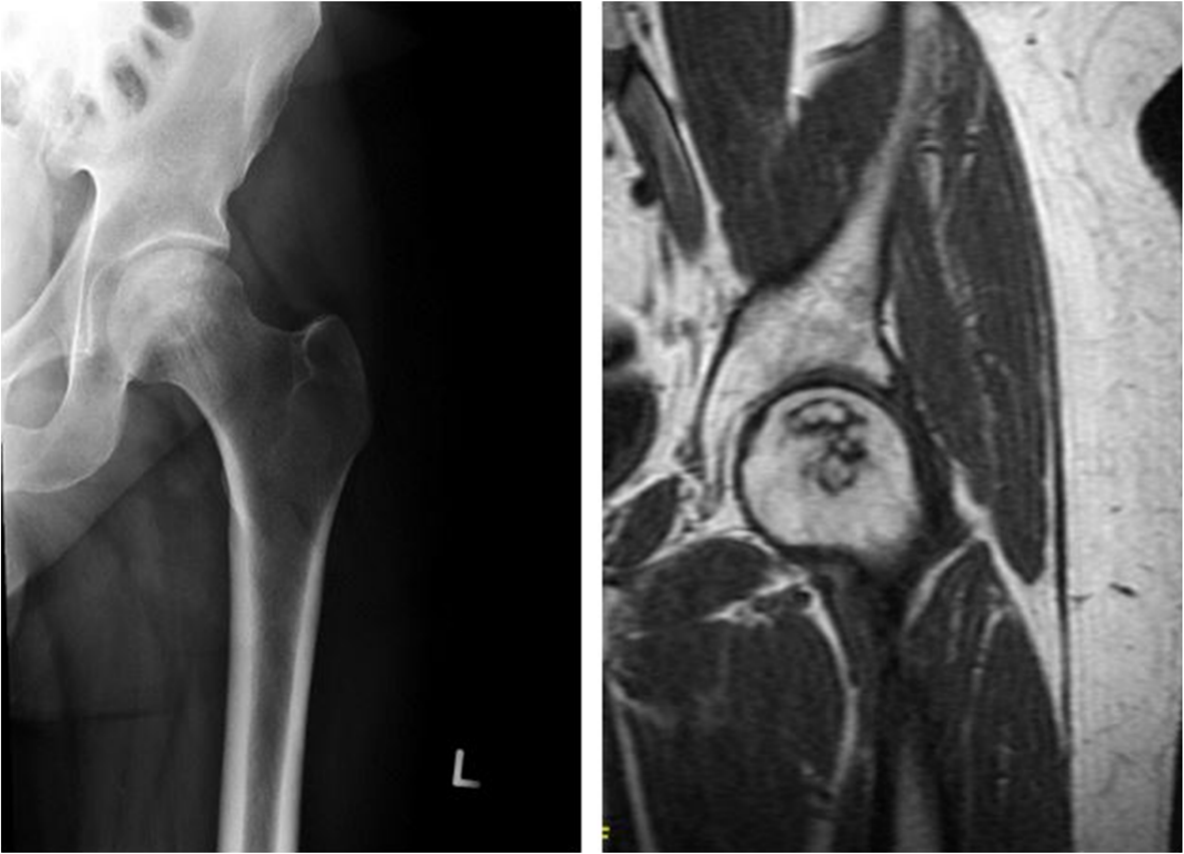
a Preoperative plain AP X-ray of left hip demonstrating a Stage IIa osteonecrotic lesion. b MRI T1-weighted coronal image of left hip shows a geographic pattern of the necrotic area
